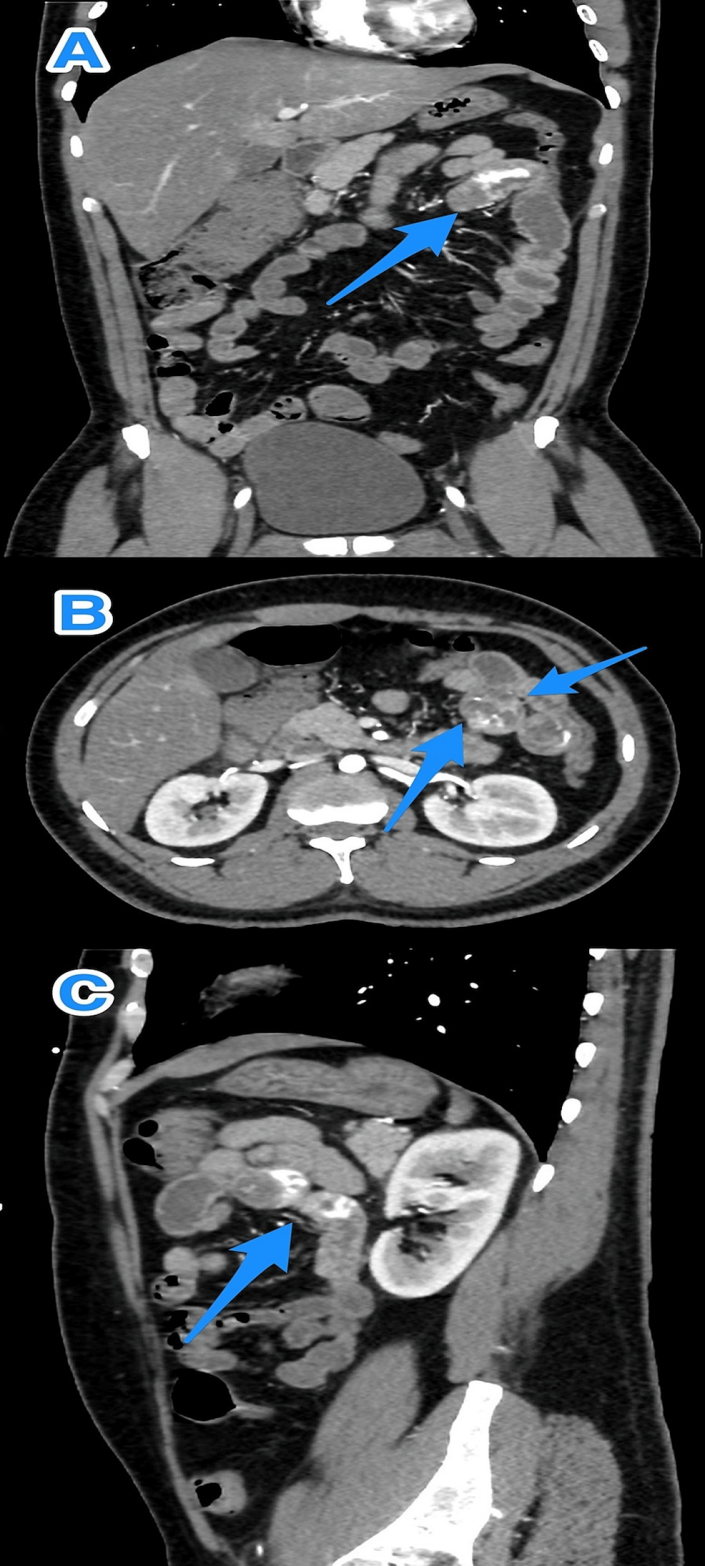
A multiview CT abdomen scan (A-C) reveals a proximal jejunal mass with extravasation (arrows) CT: computerized tomography
